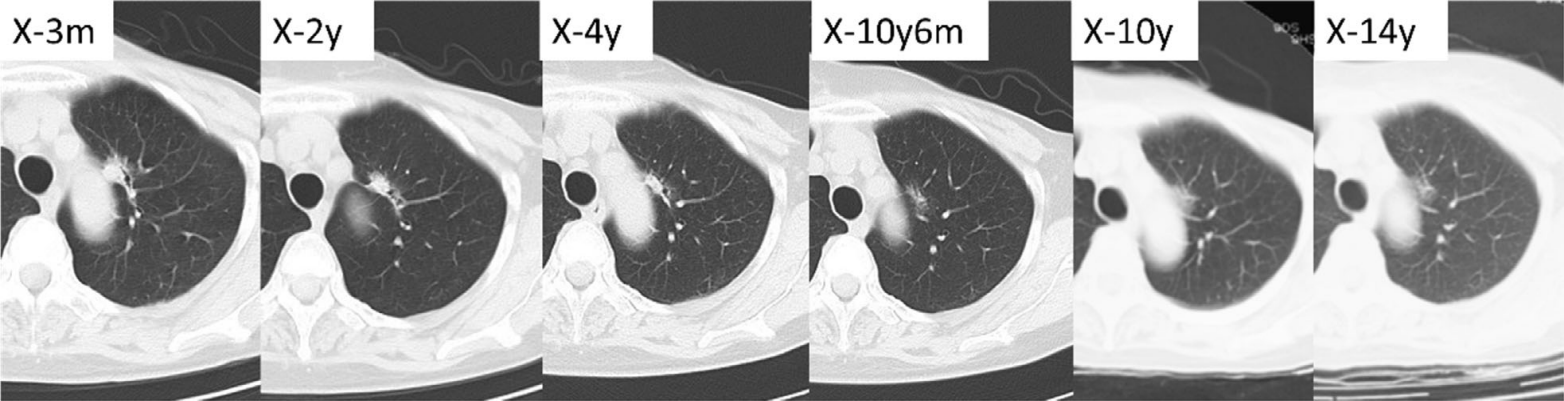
Alterations in chest CT images
